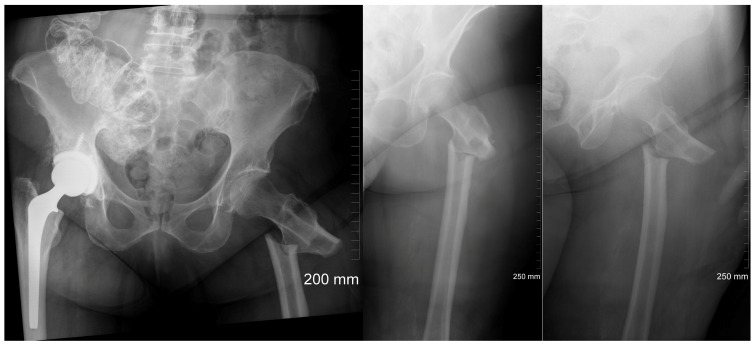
Radiographs demonstrating a transverse left subtrochanteric femur fracture with a medial spike in a 78-year-old female patient.
